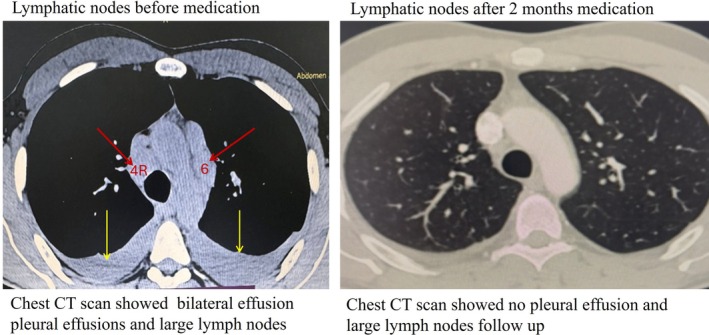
Follow‐up chest CT after 8 weeks of corticosteroid therapy showing complete resolution of pleural effusion and significant regression of lymphadenopathy. The yellow arrow indicates the previously identified pleural effusion. The red arrows indicate mediastinal lymph nodes, including station 4R (right lower paratracheal) and station 6 (para‐aortic) lymph nodes.
